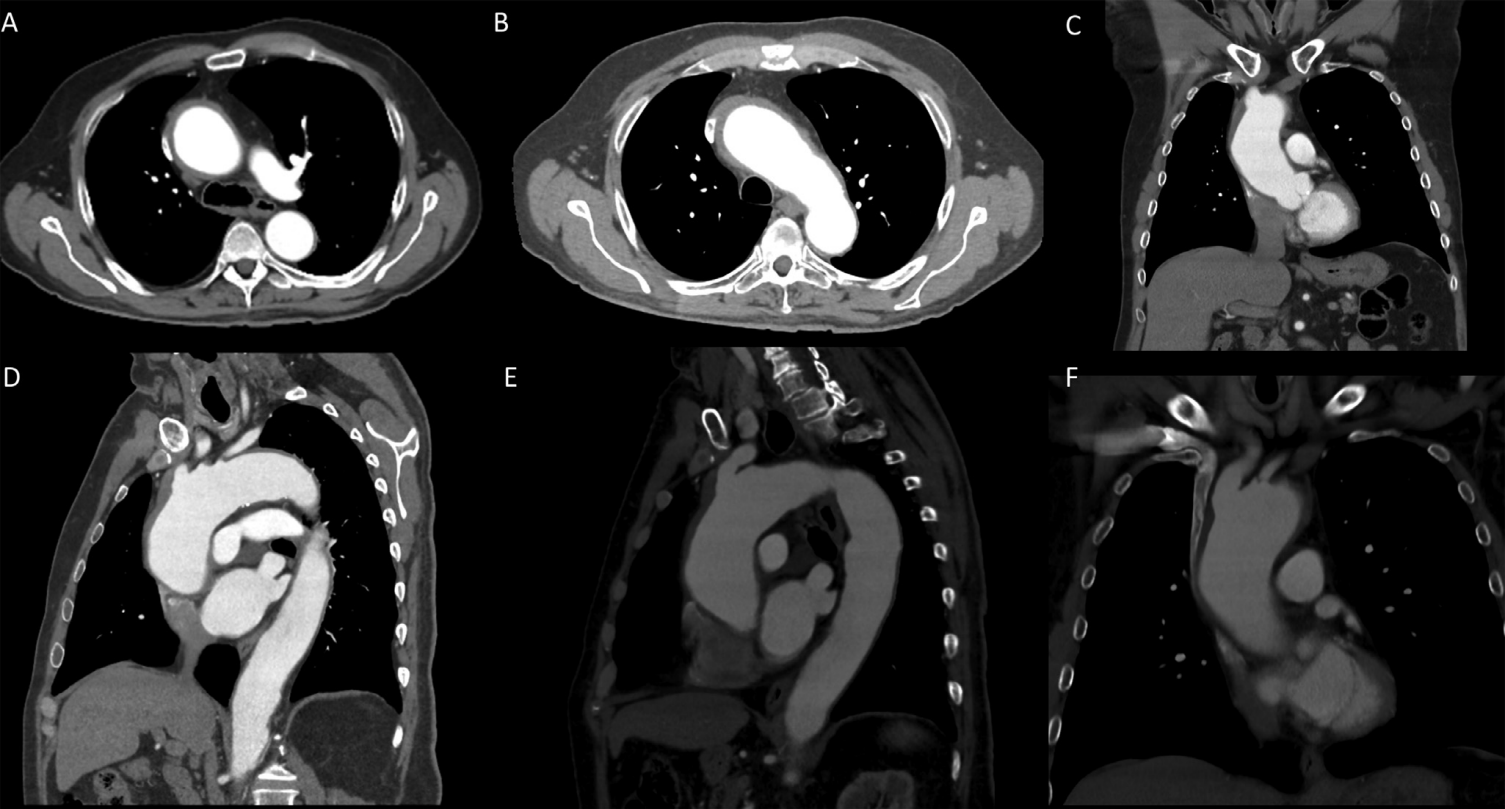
Review of outside CT chest images revealed ascending thoracic aortic aneurysm (5.3 cm in largest diameter). In addition, circumferential soft tissue attenuation thickening was noted involving the aortic root, predominately in the ascending thoracic aorta and the proximal aortic arch. A small ulceration in the aortic arch between the brachiocephalic artery and left common carotid artery was also noted. The arch vessels were normal. Constellation of these findings without significant atherosclerotic vascular disease and intimal calcification were suggestive of aortic vasculitis, specifically giant cell arteritis (GCA)
